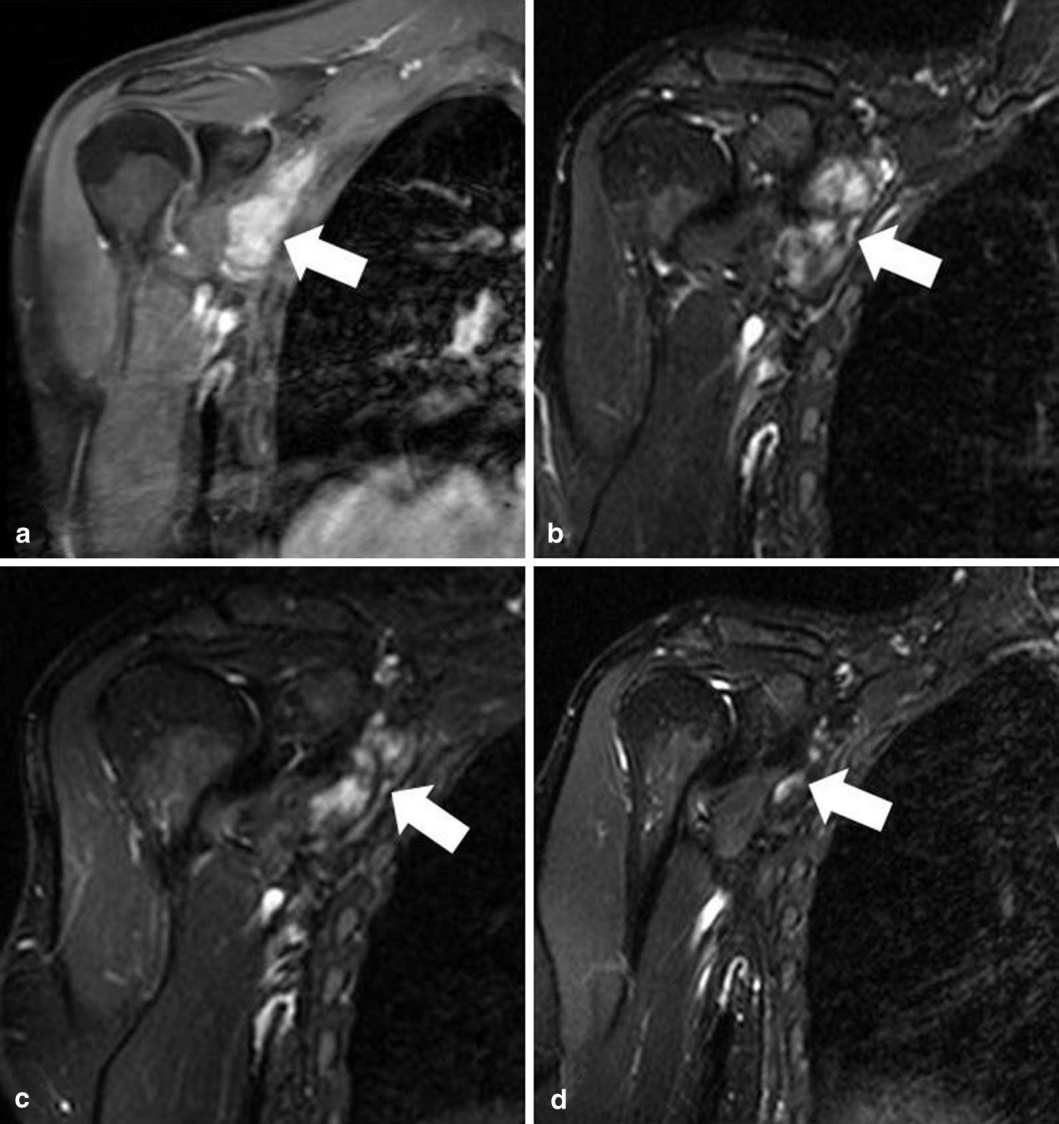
T2-weighted MRI of the right shoulder after second tumor resection and prior to bevacizumab treatment (a), as well as 6 weeks (b), 4 months (c) and 14 months (d) after treatment initiation, showing a continuous tumor shrinkage over time (*white arrows*)
